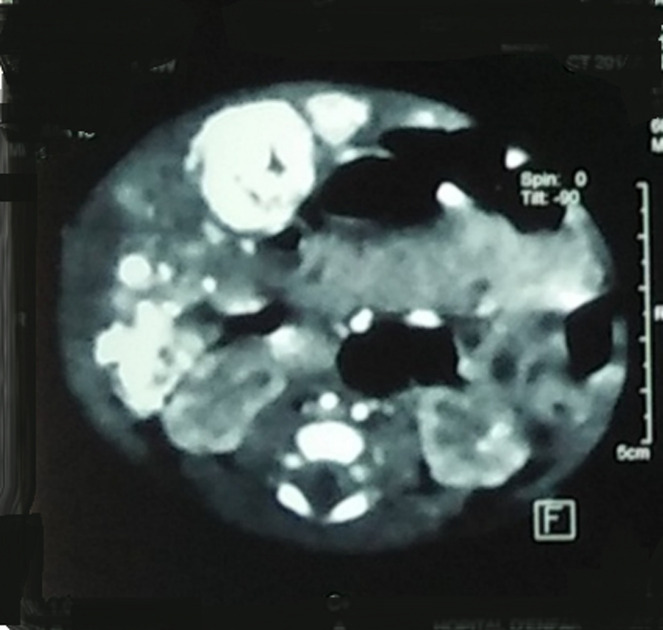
abdominal computed tomography cross-section: hepatic angiomas
